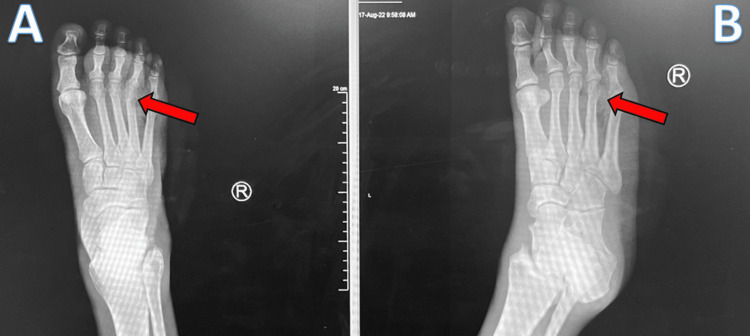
Anterior and Lateral X-Ray Images of Right Foot Anterior (A) and lateral (B) view of right foot demonstrating extensive subcutaneous gas along the fibular and dorsal aspect of the forefoot (red arrows).
